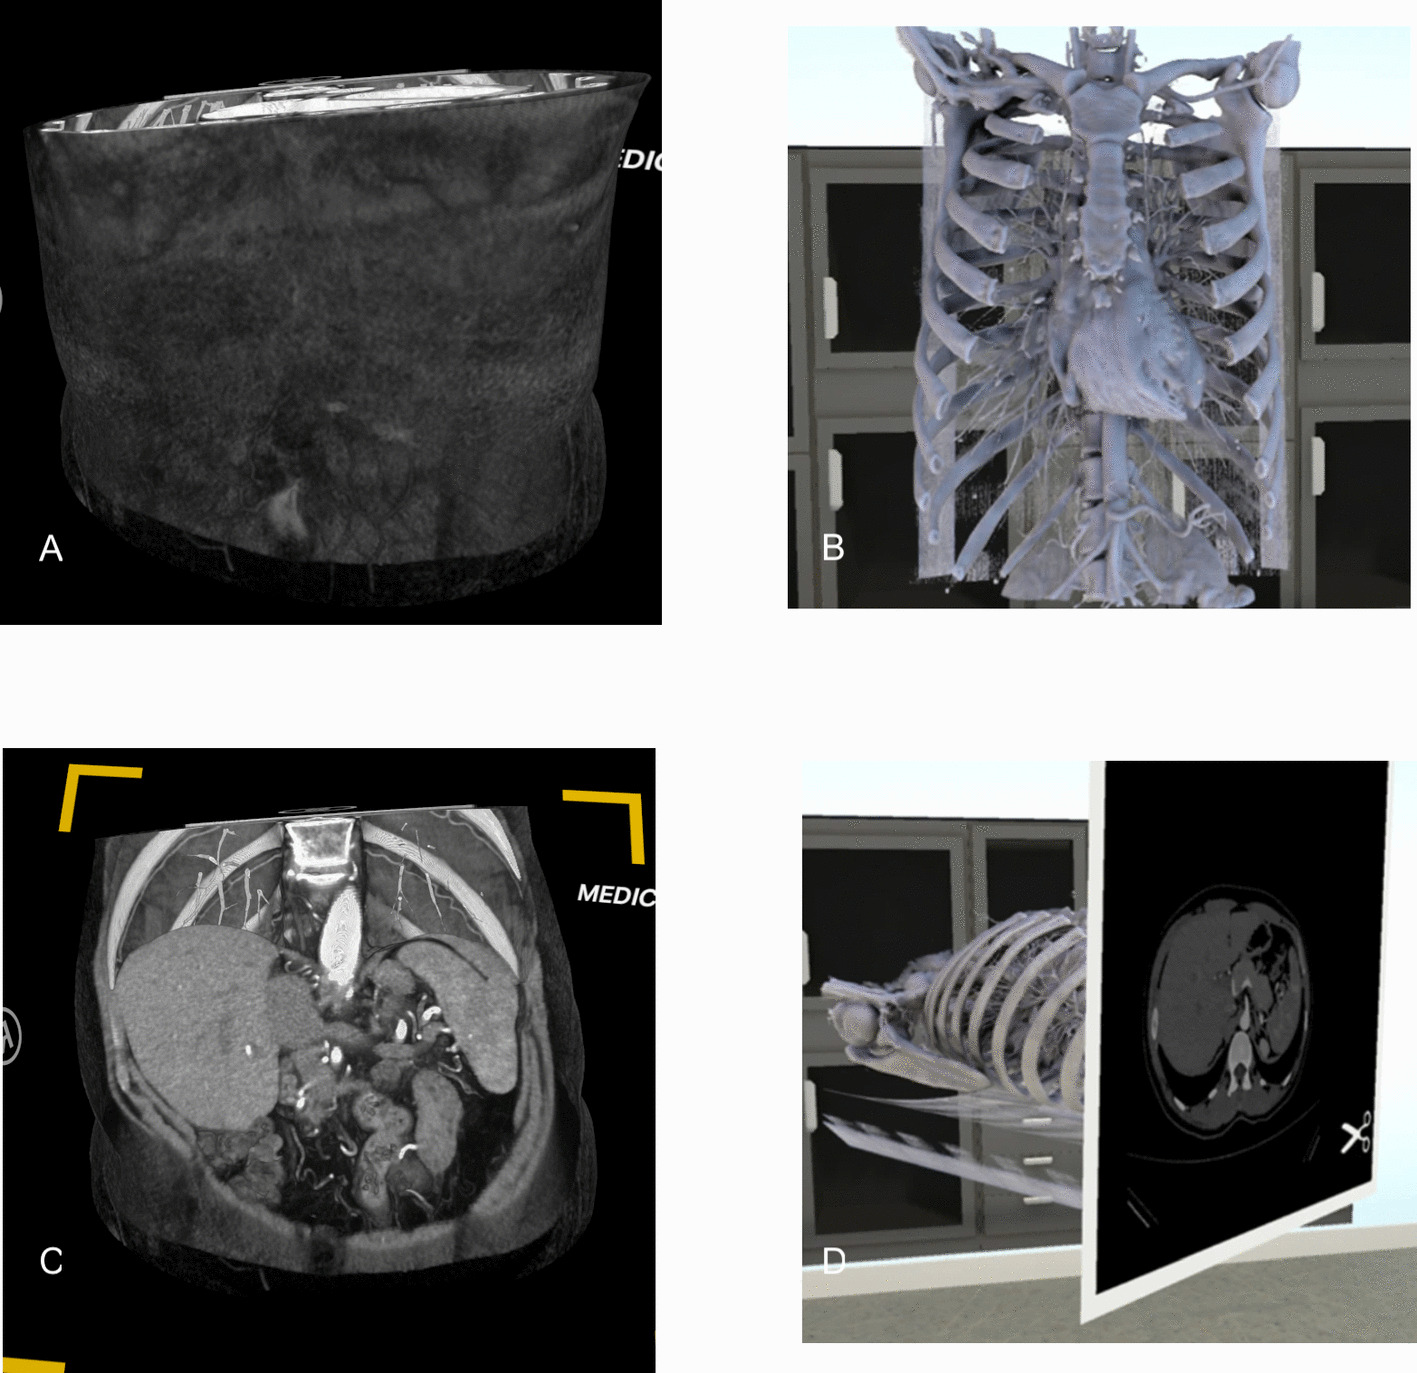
Comparison of visualization approaches: volumetric rendering versus hybrid vascular-skeletal display. Representative images comparing two distinct VR implementation strategies for pancreatic tumor assessment. Left panels show the volumetric rendering approach used in this study: coronal view (A) and axial view (C) displaying abdominal CT with minimal opacity adjustments approximating CSI abdominal windowing, maintaining visualization of all soft tissue structures including pancreas, liver, and bowel. Right panels (B, D) demonstrate the hybrid approach described by Kunz et al., displaying exclusively isolated osseous structures and contrast-enhanced vessels with integrated cross-sectional cutting planes. Original image cropped and adapted from Kunz JM, Maloca P, Allemann A, et al. Assessment of resectability of pancreatic cancer using novel immersive high-performance virtual reality rendering of abdominal computed tomography and magnetic resonance imaging. Int J Comput Assist Radiol Surg. 2024;19:1677–1687. Licensed under CC BY 4.0 (http://creativecommons.org/licenses/by/4.0/). Image cropped and combined with author's original material. CT indicates computed tomography; VR, virtual reality
